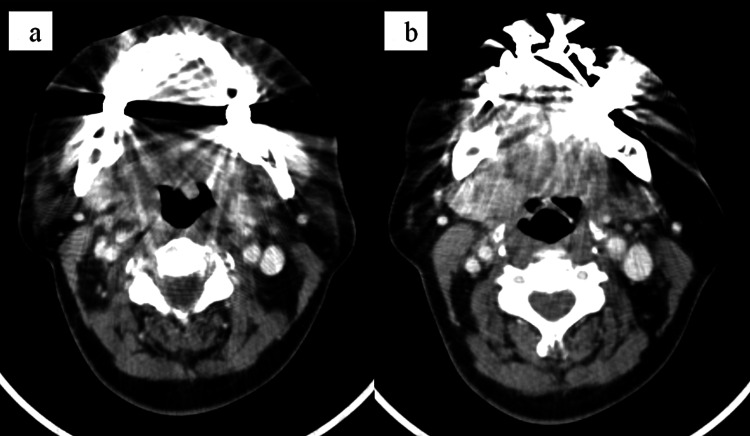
Radiological findings about two years and two months after the start of radiation therapy. (a, b) The axial computed tomography images. The cervical lymph node metastases had shrunk and were no longer identifiable.
